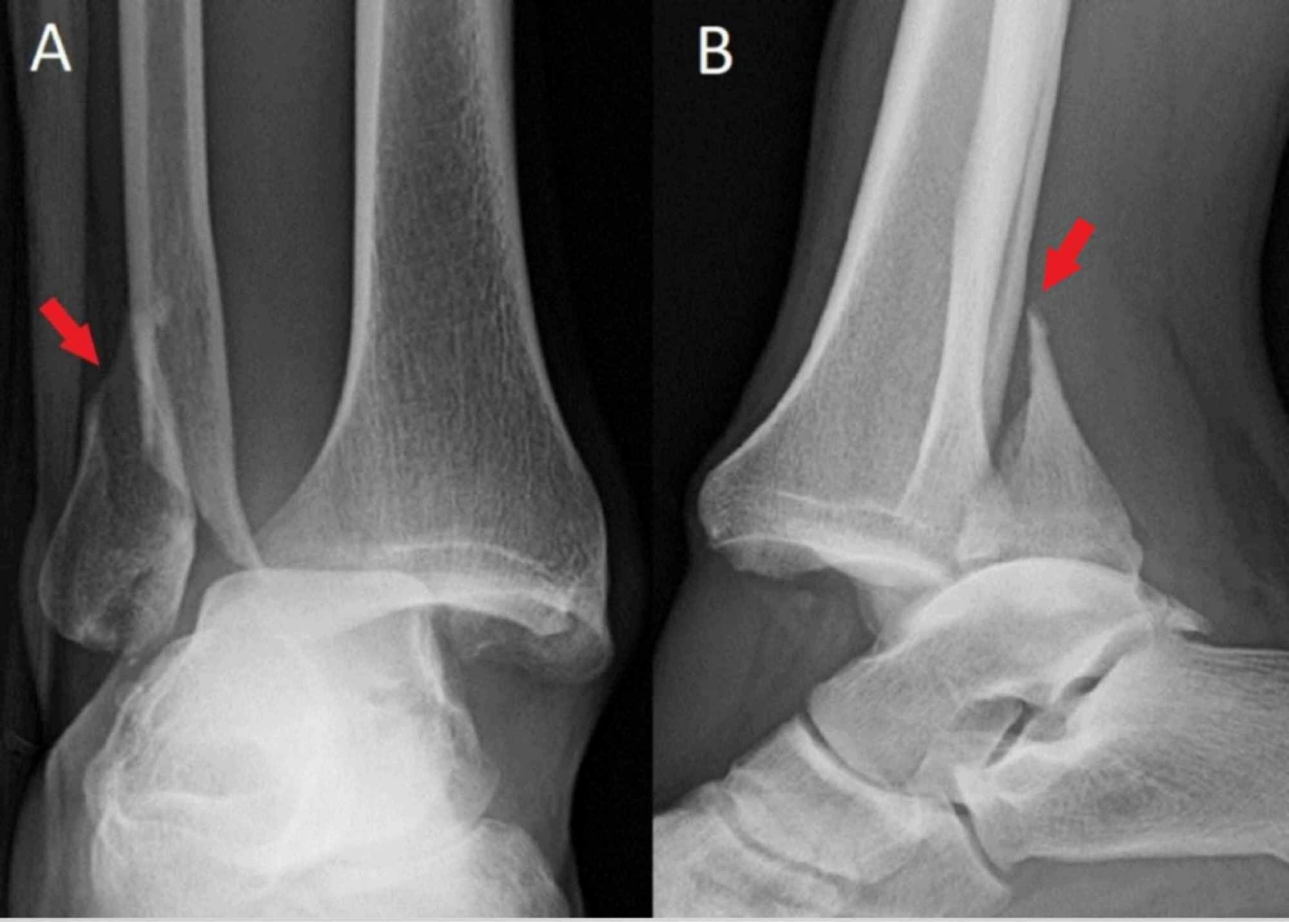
Initial injury radiographs Anteroposterior (A) and lateral (B) radiographs of the initial injury show tibiotalar dislocation and fracture of the lateral malleolus (red arrows). Initially it was difficult to assess the location of the proximal fragment of the fibula in relation to the posterior tibial tubercle due to the external rotation of the talus relative to the tibia.
